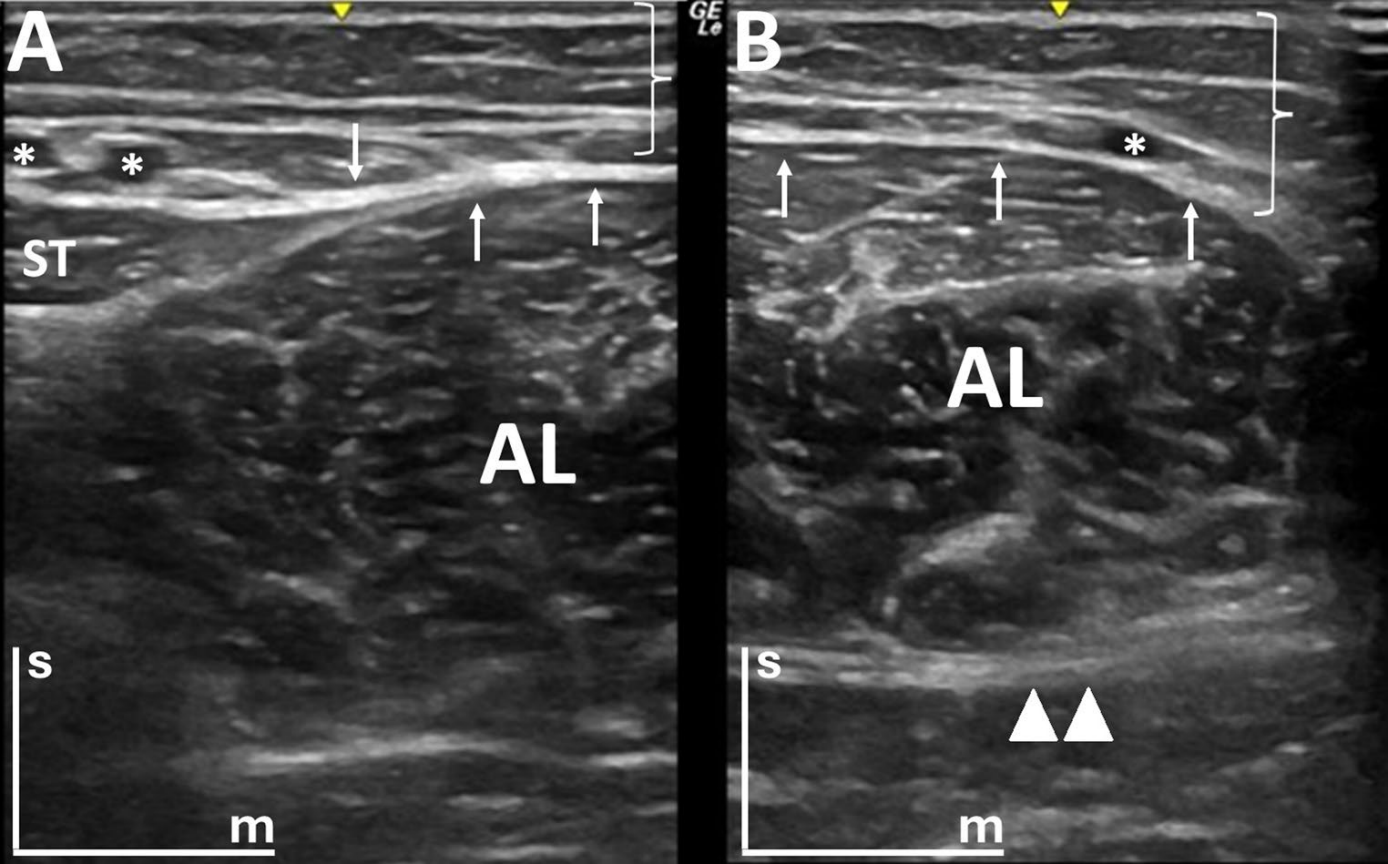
Ultrasound images of the proximal thigh in the transversal section (B-mode). The skin positioned at the top of each image is indicated by the bars (“s” for superficial and “m” for medial). White arrows highlight the fascia lata, and the investing fascia of the thigh. Brackets denote the tela subcutanea (superficial fascia). A) This image represents the lateral cut of the adductor longus, where two veins from the great saphenous system are visible (marked with white asterisks); and B) This image represents the medial cut of the adductor longus, where only one vein is shown (marked with white asterisks), in addition to the anterior branch of the obturator nerve (represented with a double triangle, ▲▲). AL: adductor longus muscle; ST: sartorius muscle
